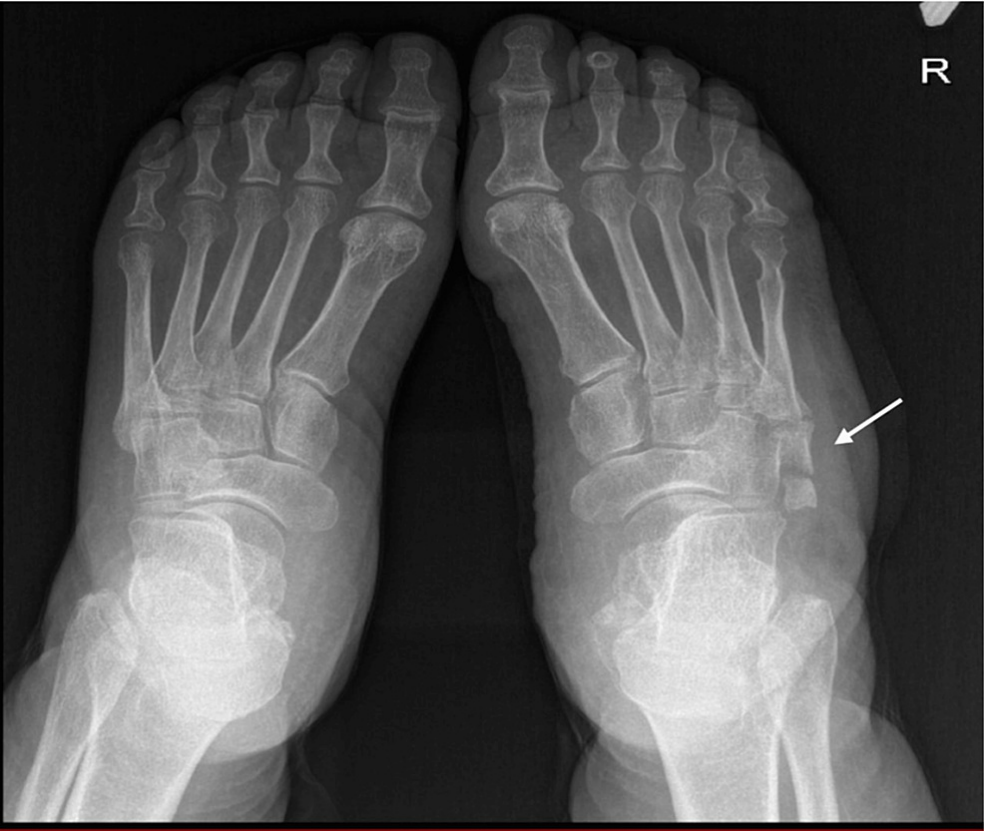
Bilateral foot X-ray anteroposterior view - accessory bone in the base of the right fifth metatarsal bone (arrow).
